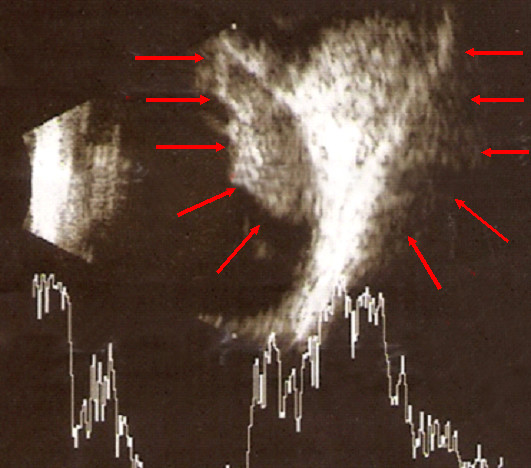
Transverse B-scan ultrasound of superior posterior pole, left eye, shows choroidal mass (red arrows).
